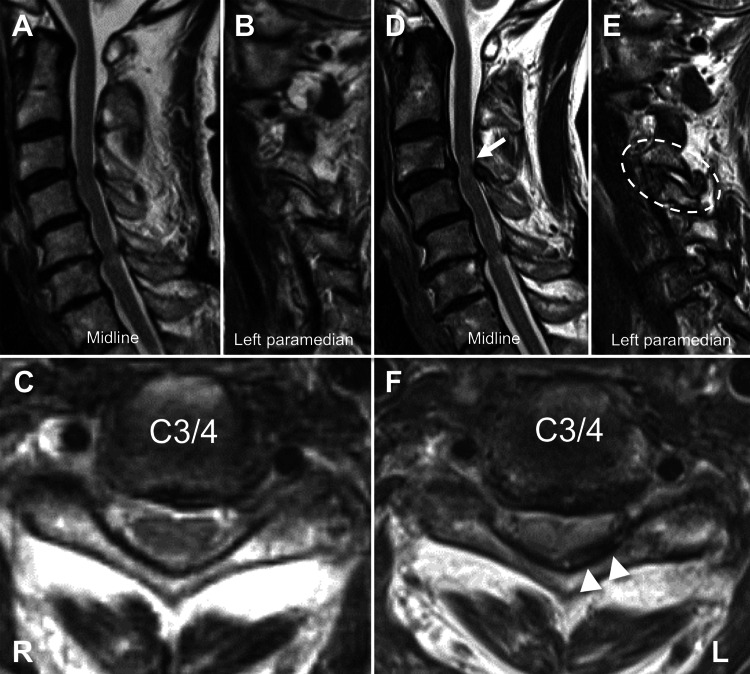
Preoperative T2-weighted magnetic resonance images 15 months (A–C) and just (D–F) before surgery. Sagittal images demonstrate increased intramedullary signal intensity (arrow) and enlargement of the intra-articular gap in the left C3-4 facet joint (circle). Axial images indicate the emergence of asymmetrical ligamentum flavum hypertrophy (arrowheads).
